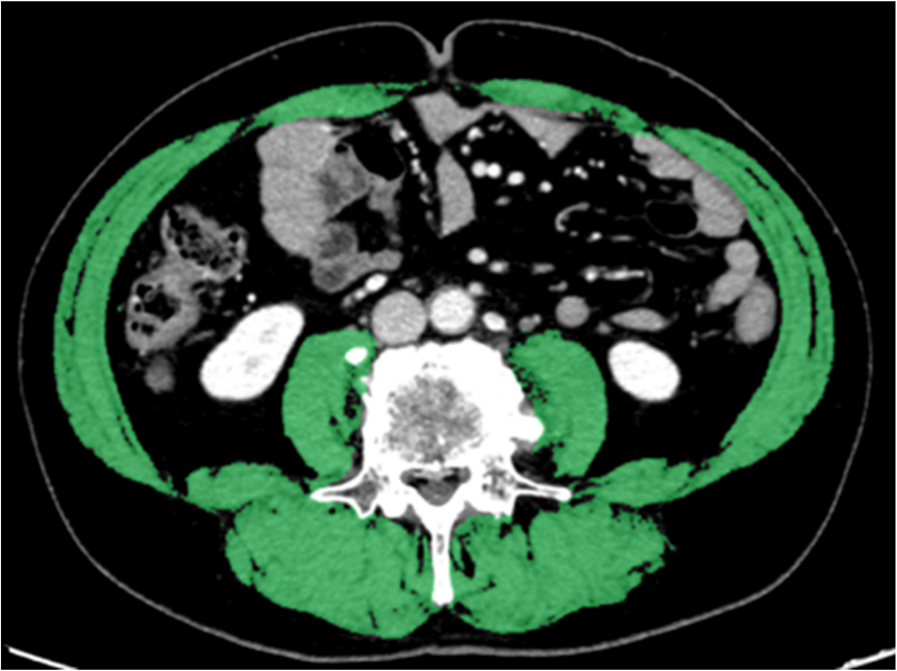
Measurement of skeletal muscle mass in patients with unresectable gastric cancer. Axial computed tomography slice of the third lumbar vertebra. Green areas indicate skeletal muscle mass. Abbreviations: SMM, skeletal muscle mass; UGC, unresectable gastric cancer
